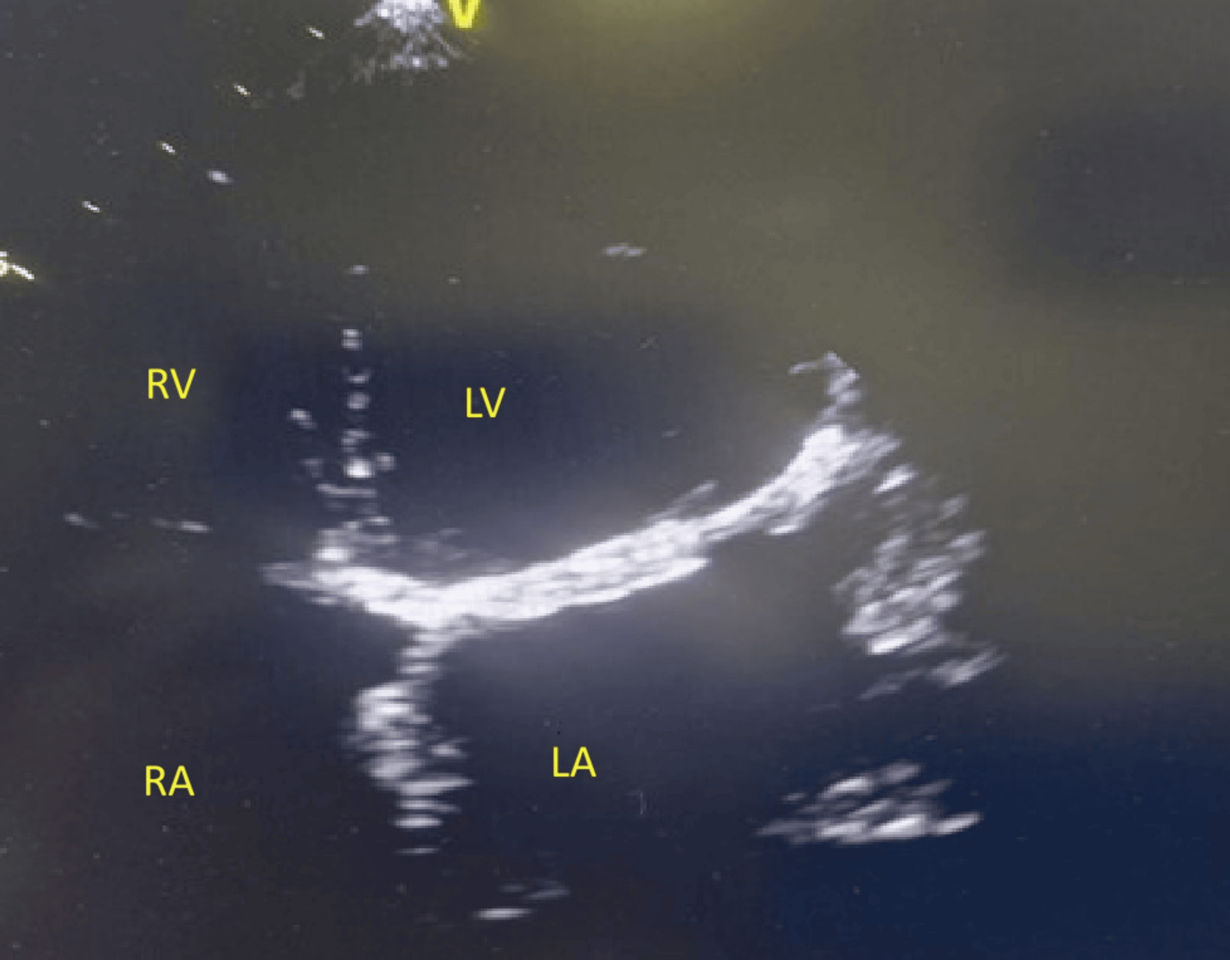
Echocardiography after second thrombolysis showing resolution of thrombus in apical four chamber view (4C) LA - left atrium; LV - left ventricle; RA - right atrium; RV - right ventricle
